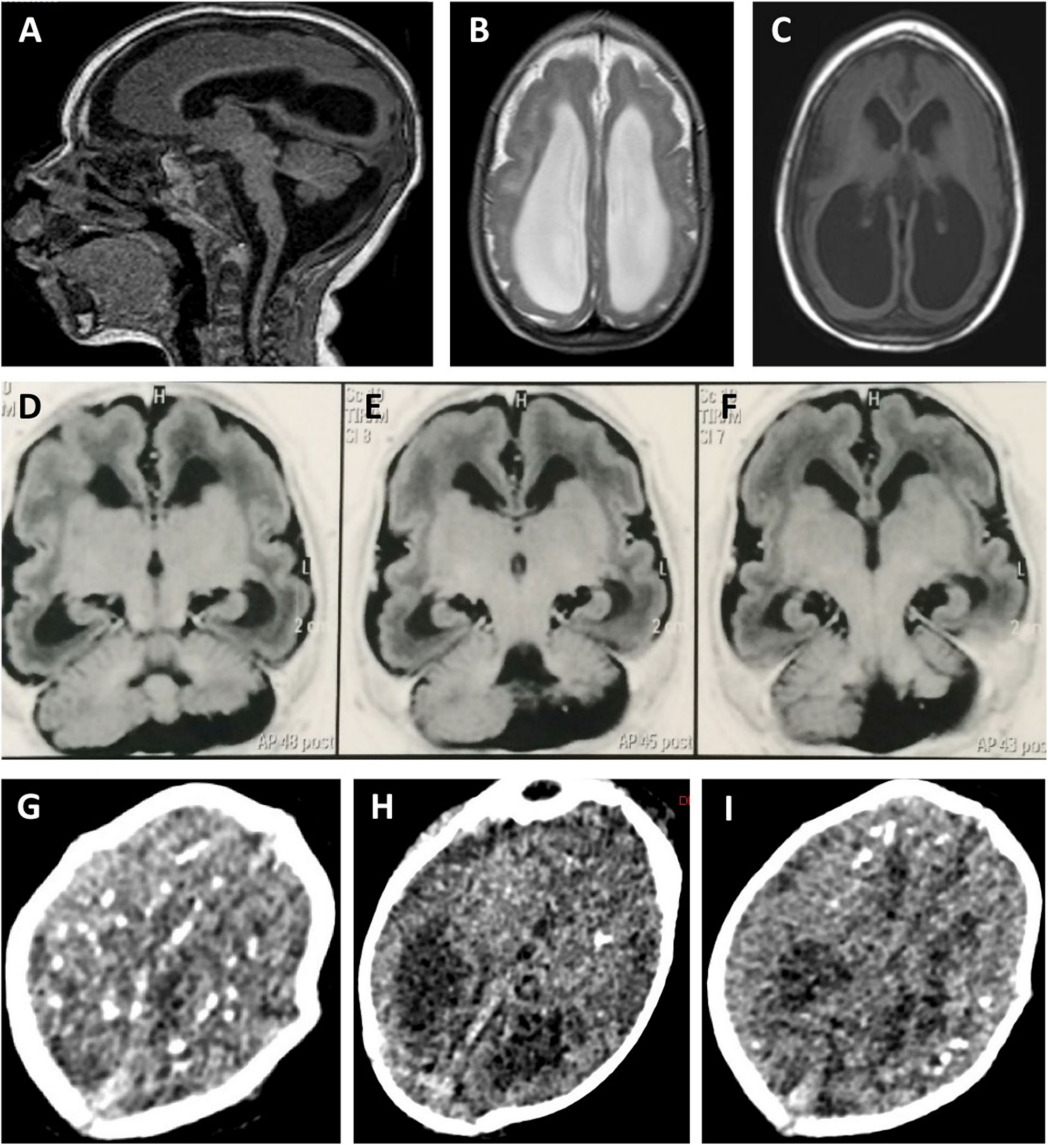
Brain Imaging after birth. a MRI Sagittal T1 weighted image: hypogenesis of the corpus callosum, enlarged cisterna magna, and ventriculomegaly. b MRI Axial T2 weighted image: simplified frontal gyral pattern, ventriculomegaly. c Axial T1 weighted image: pachygyria/lissencephaly in the frontal lobe, ventriculomegaly. d, e, f MRI Axial T1 weighted image: simplified frontal gyral pattern, ventriculomegaly, cerebellum hemisphere hypoplasia. g, h, i Axial non-contrast CT image: multiple bilateral calcifications in the junction between cortical and subcortical white matter, ventricular enlargement
